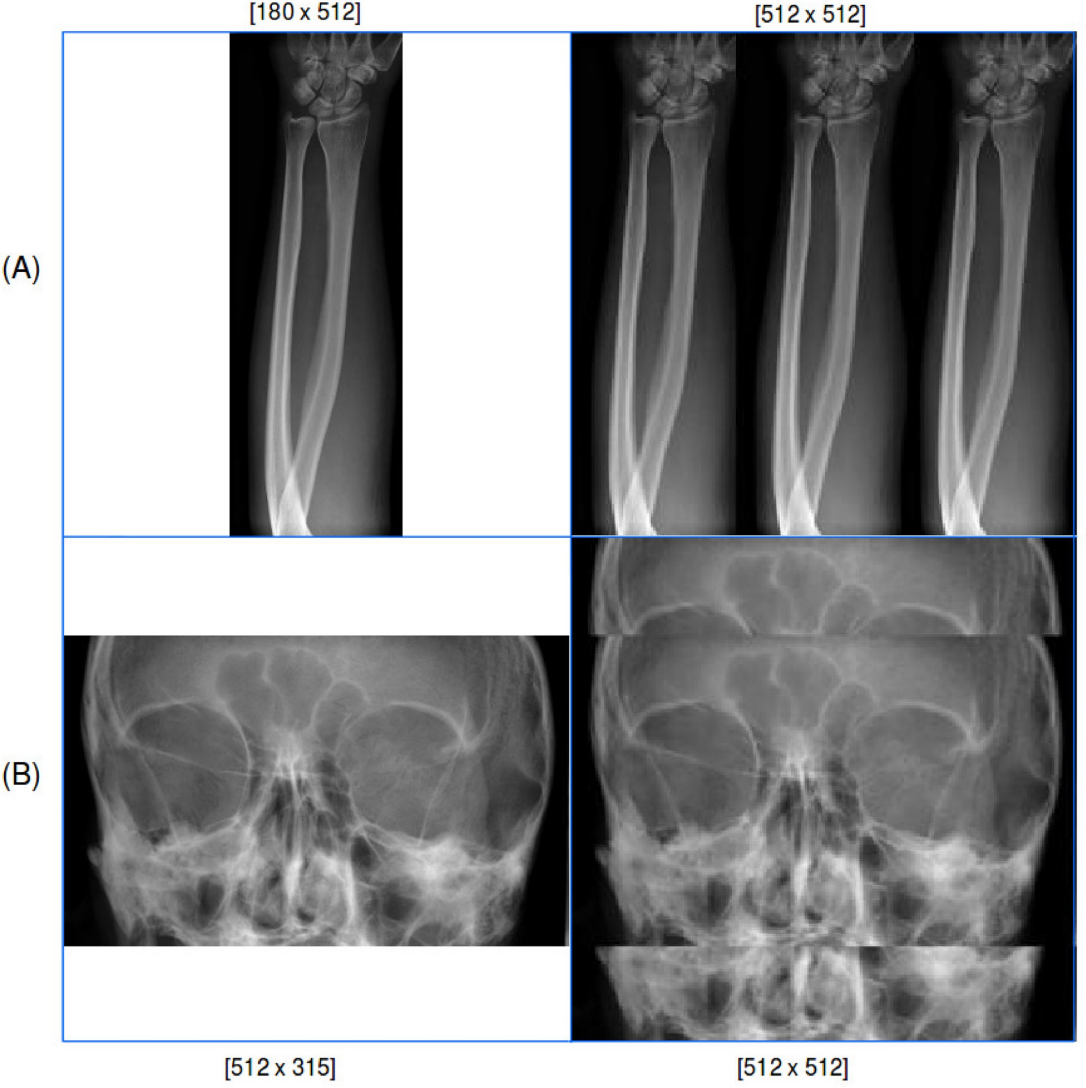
Resized radiographs by padding input images to the defined width and height size [512 x 512]. (A) shows horizontal and (B) vertical padding. The radiographs were randomly chosen from the ImageCLEF 2009 Medical Annotation Task Training Set. Modified from [21] under a CC BY license, with permission from [RWTH Aachen], original copyright [2009].
